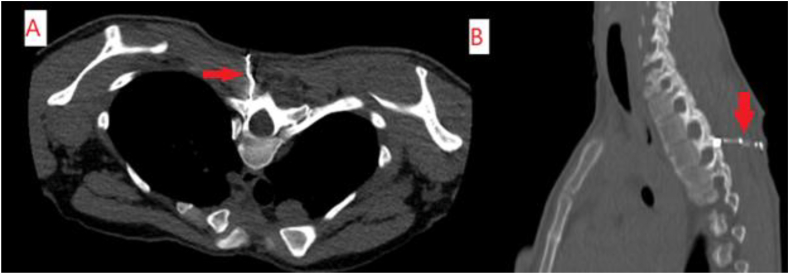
Preoperative CT-guided placement of a metallic localization wire (red arrows) into the left T4 pedicle to facilitate accurate intraoperative level identification. (A: axial cut; B: sagittal cut).
